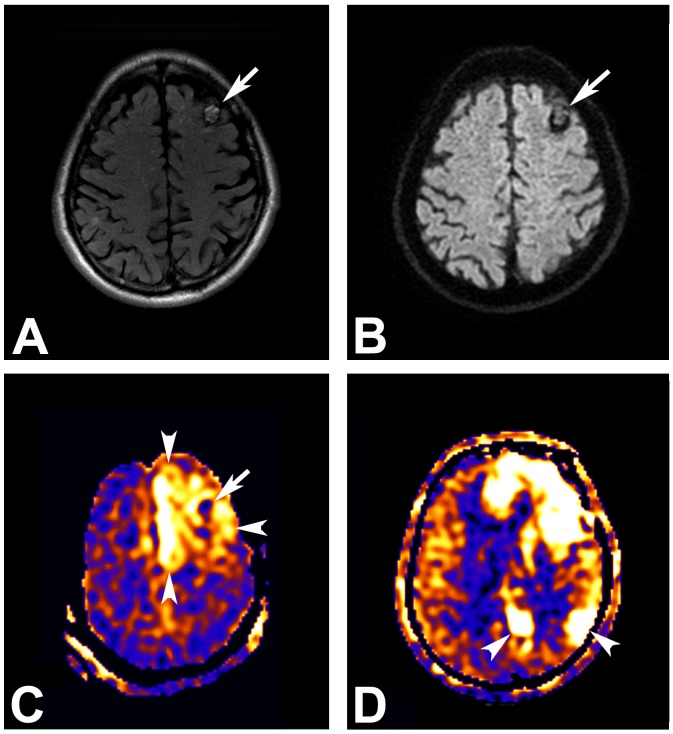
A 71-year-old man with underlying cavernous malformation who presented to the emergency department with a seizure. (A) T2 FLAIR MR image demonstrates a cavernous malformation (arrow) with a hemoderin rim and typical popcorn ball appearance secondary to multiple locules containing hemorrhage at the left frontal lobe. (B) Diffusion-weighted image shows no abnormal signal change around the cavernous malformation (arrow). (C) Arterial spin labeling perfusion-weighted MR image, however, reveals prominent perilesional hyperperfusion (arrowheads) surrounding the cavernous malformation (arrow) at the left frontal lobe. (D) Additional hyperperfusion foci (arrowheads) are also noted at the left parietal lobe.
